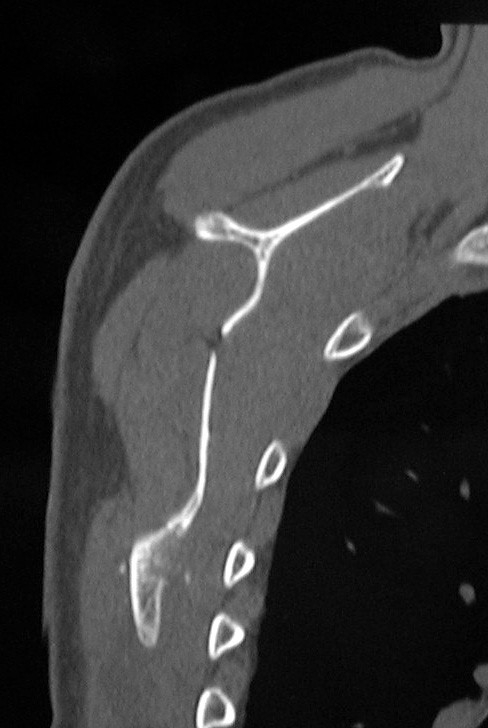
Postoperatively, CT reveals the complete resection the tumor.
